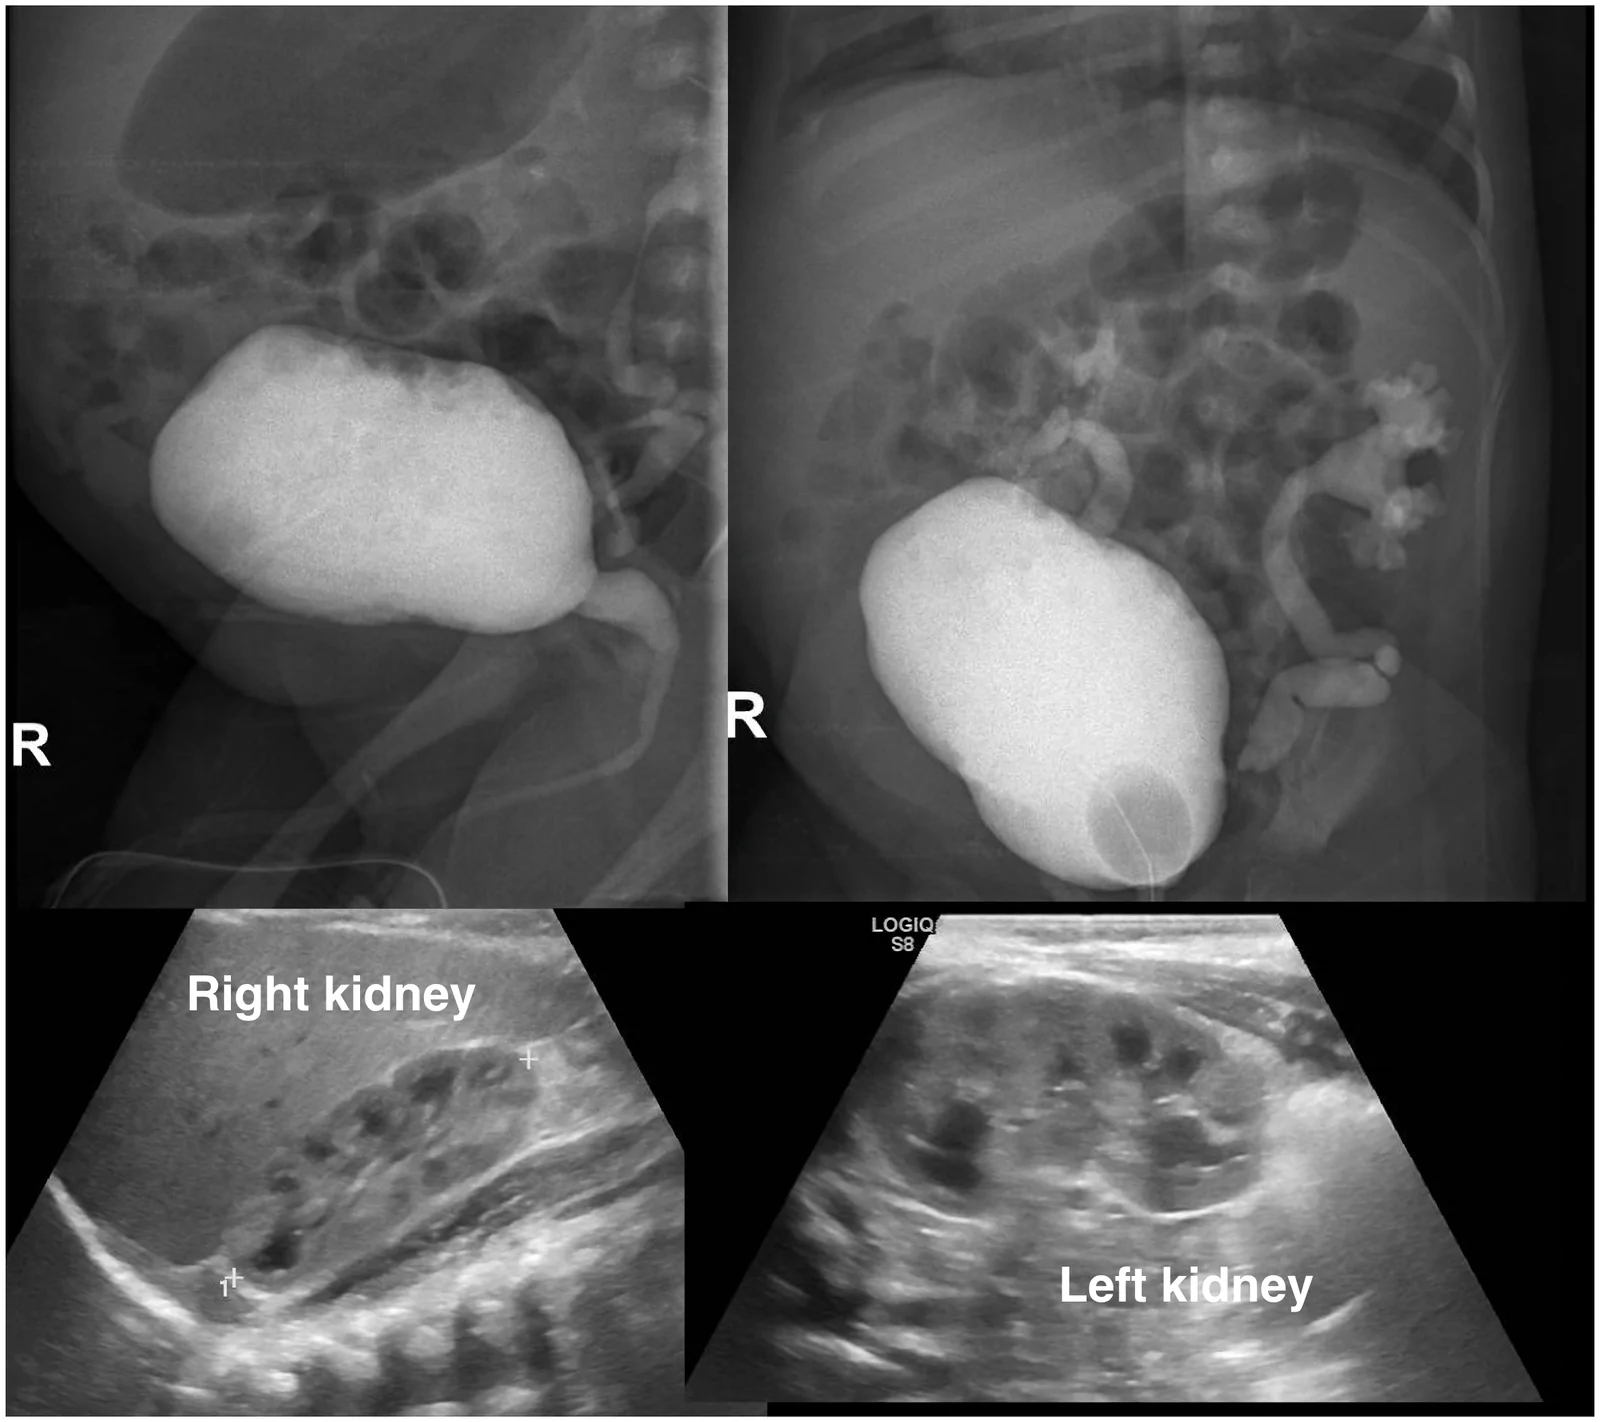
Voiding cystography with high-grade vesicoureteral reflux. Ultrasound on the 7th day of life.
